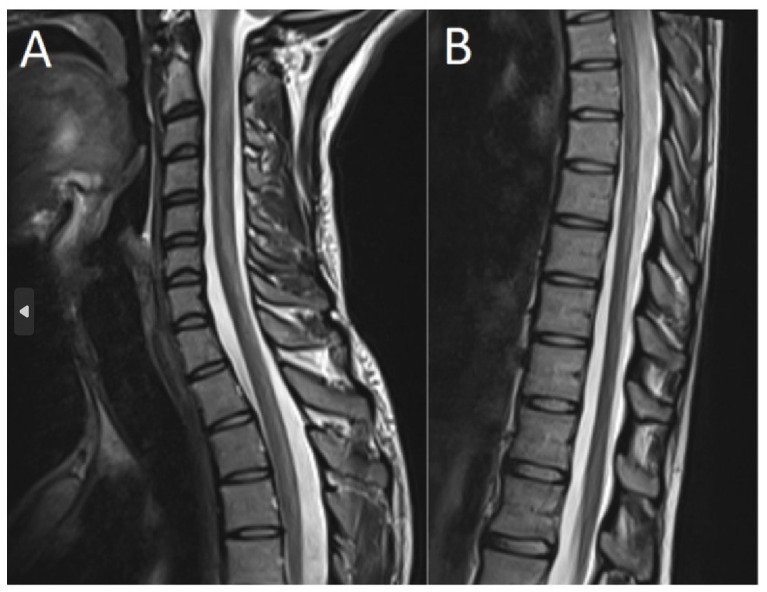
T2-weighted contrast-enhanced spine MRI shows subacute combined degeneration. Contrast enhancement was observed in the cervical (A) and thoracic (B) spinal cord. Investigations were performed two days after admission.
